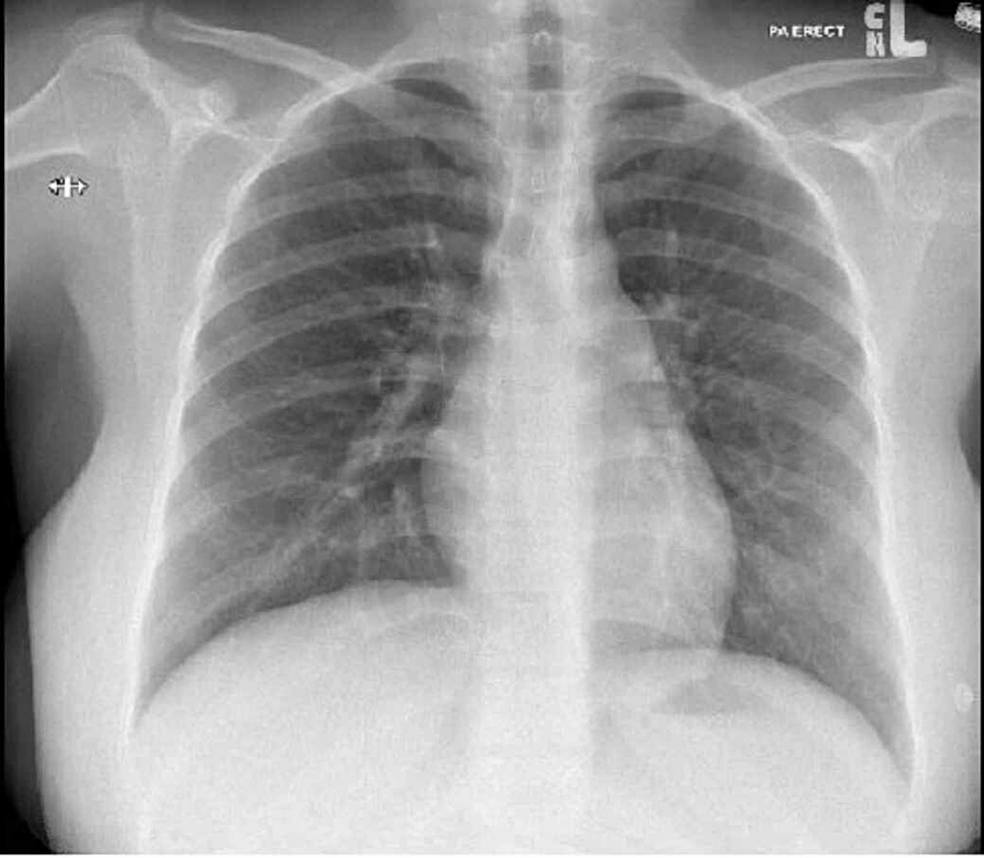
Erect posteroanterior chest X-ray showing resolution of pulmonary edema after diuresis with intravenous furosemide.
